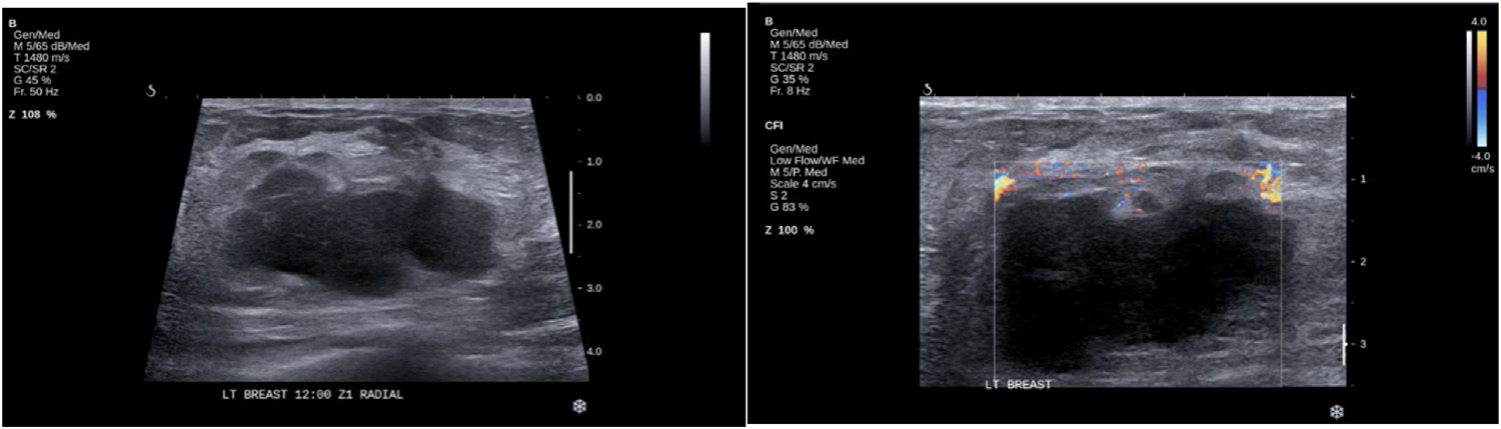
Ultrasound shows a heterogenous mainly hypoechoic irregular microlobulated solid large mass at 12 o'clock anterior third measures 4.9 × 3.2 × 4.8 cm with peripheral vascularity .
